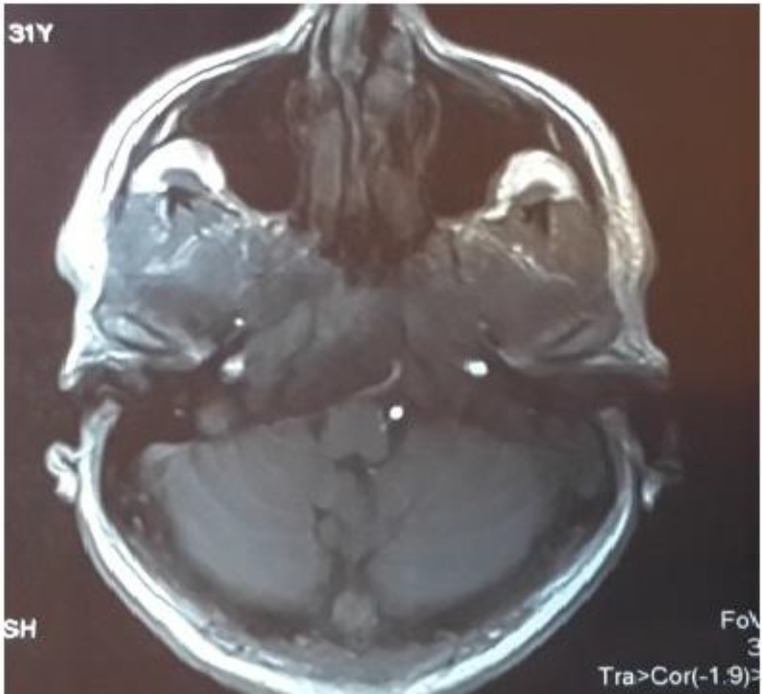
Brain MRI axial T1WI without Gad showing left mastoid air cell opacification and involvement of the left petrous apex
